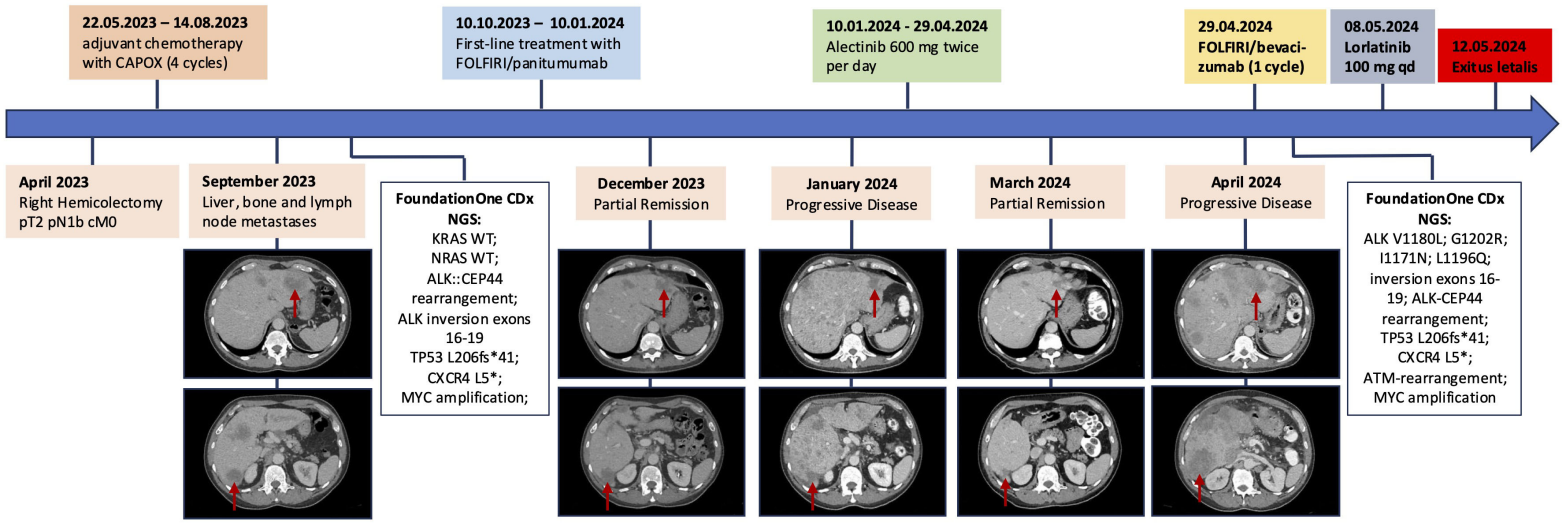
Timeline of the patient’s clinical history, summarizing therapeutic interventions, computed tomography findings with focus on liver metastases, and molecular profiling results at two time points. The red arrows each point to the liver metastases in segment II and segment VI, respectively.
